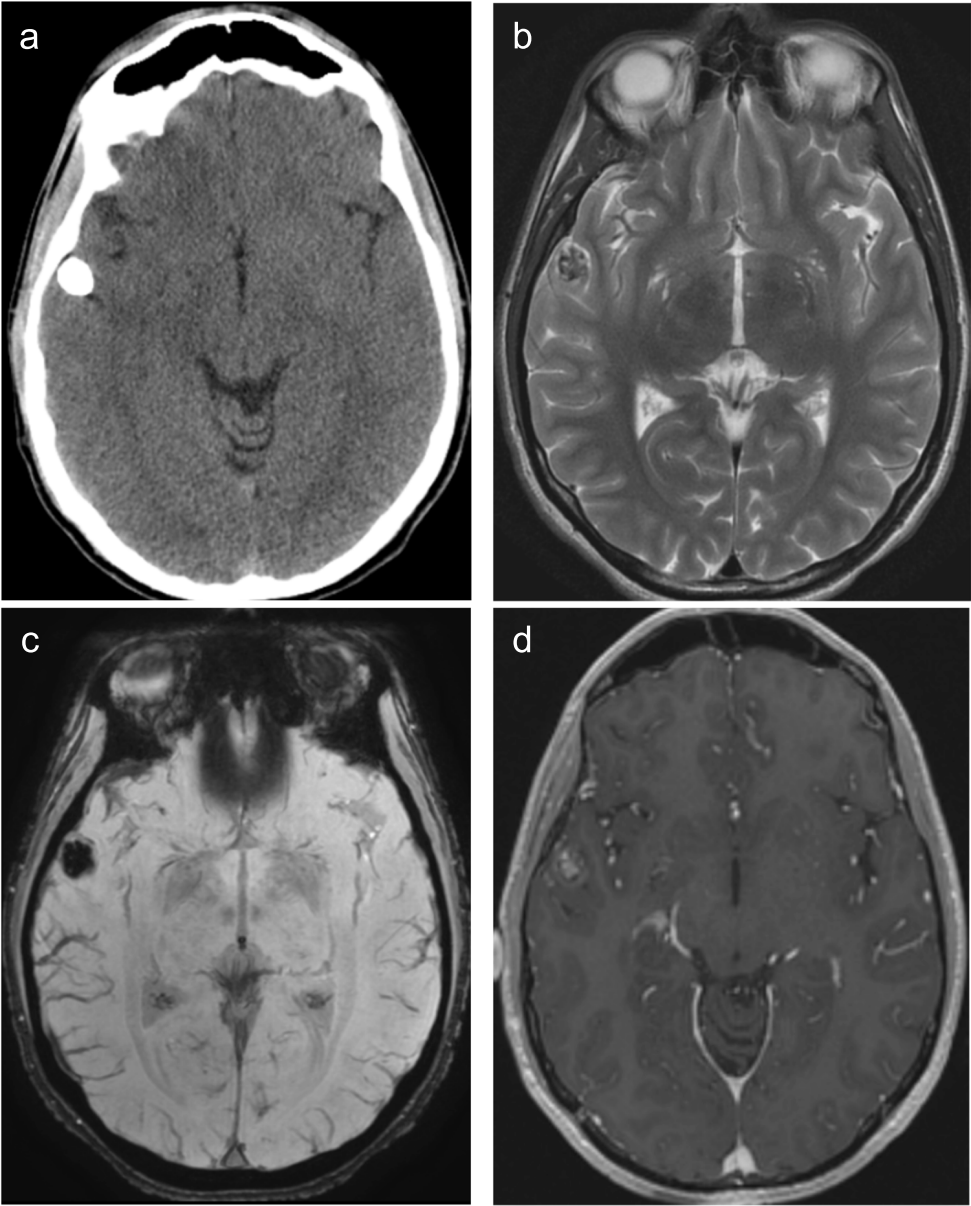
Polymorphous low-grade neuroepithelial tumor of the young (PLNTY) with *BRAF V600E* mutation in a 29-year-old male with new seizure. Axial CT (a) image shows a densely calcified lesion in the right temporal lobe. Axial T2-weighted (b), SWI (c), and T1-weighted contrast-enhanced (d) MR images reveal a circumscribed, cortically based, T2-heterogeneous signal mass in the right temporal lobe with blooming artifact (SWI) and nodular internal enhancement
